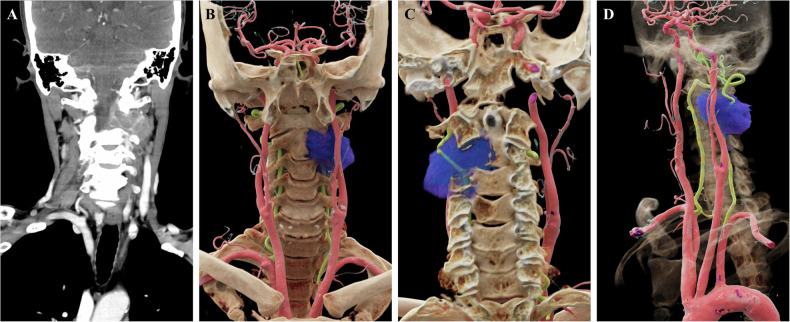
Images of a 68-year-old male with neck pain. A The coronal cervical CT image reveals bone destruction at the C2-C3 vertebral level, accompanied by the formation of a surrounding soft tissue mass. B–D Multi-angle CR images, with their superior depth perception capabilities, authentically depict the anatomical relationships among the tumor, bone, and blood vessels (blue: tumor; red: carotid artery; green: vertebral artery). The images clearly demonstrate that the V3 segment of the left vertebral artery is tightly encased by tumor tissue
